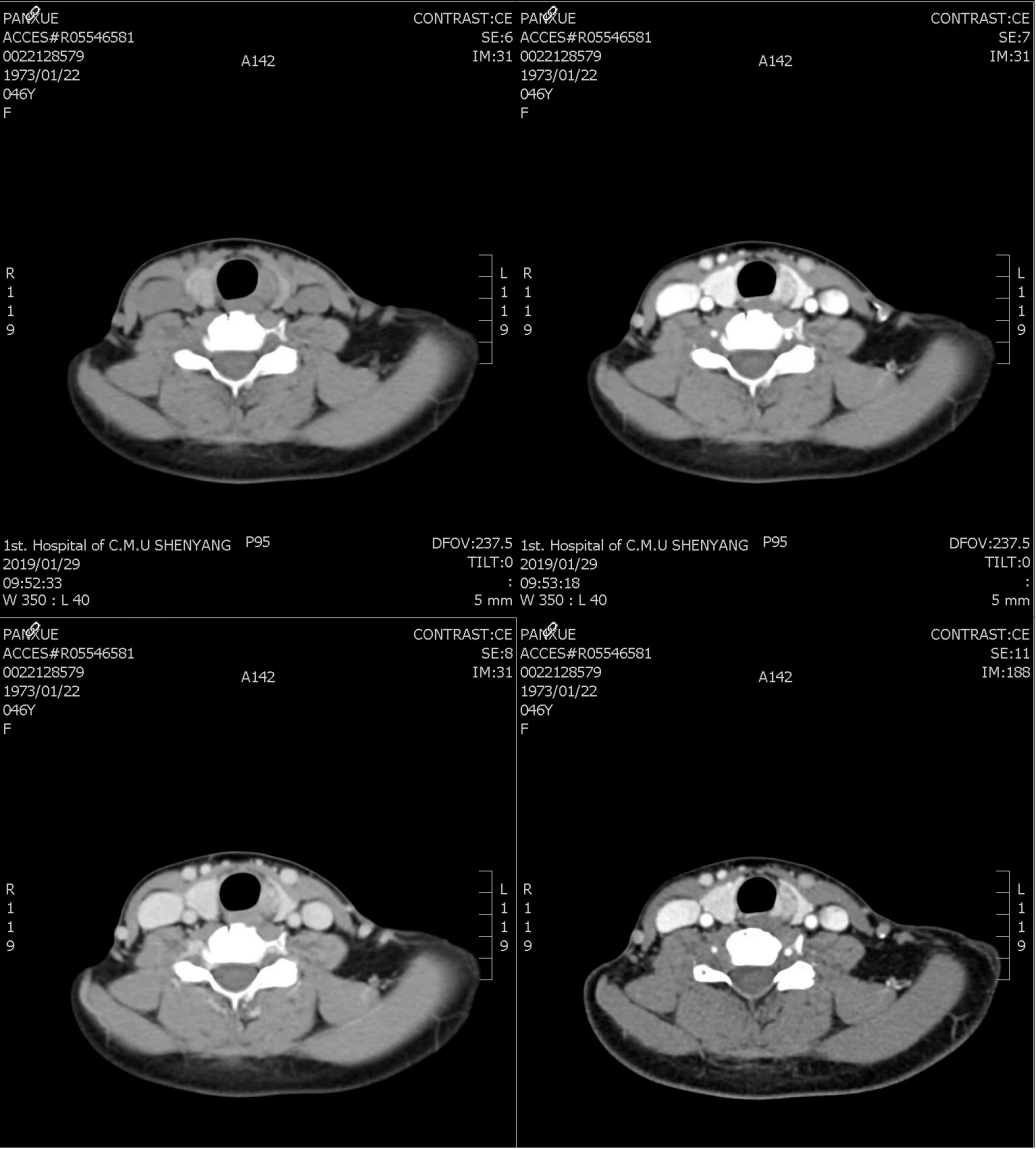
Enhanced CT image of atypical parathyroid adenoma in the neck.
